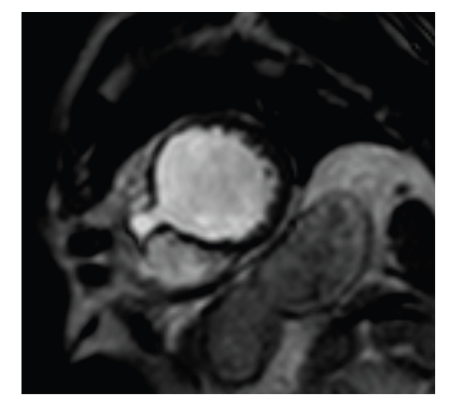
Short axis MRI shows the hemodynamically significant IVSD.
